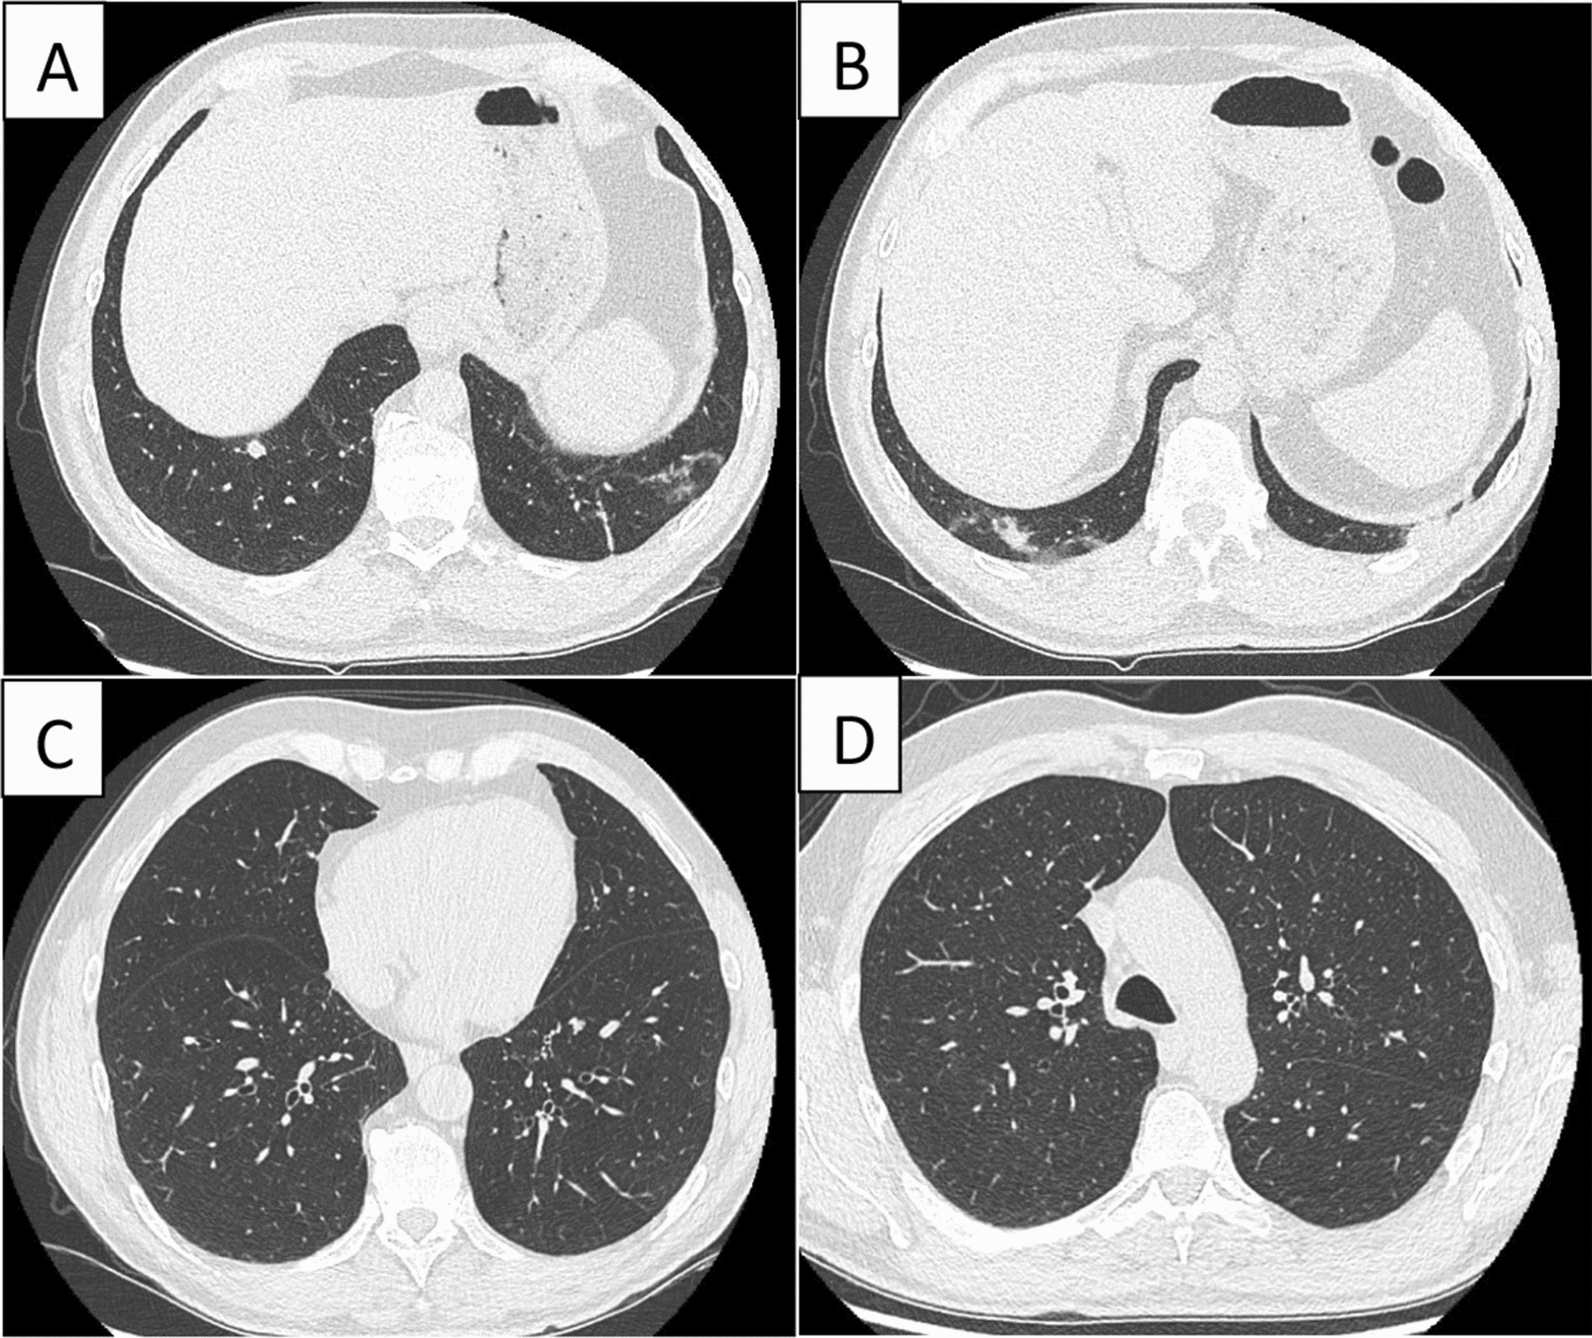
Chest CT scan. A right 8 mm juxta-diaphragmatic nodule. B right and left basi thoracic bronchiolitis. C, D Medium and upper sections of the lungs without anomalies
